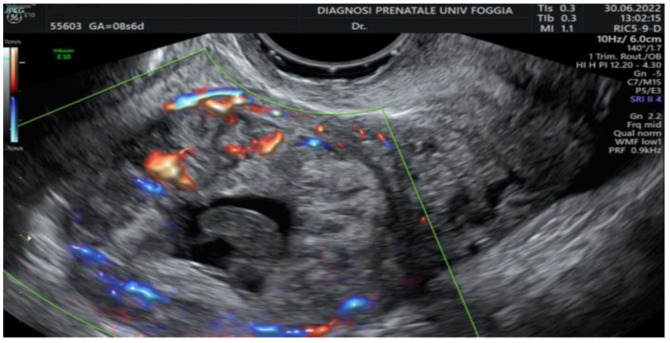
Transvaginal ultrasound control performed on day 15.
